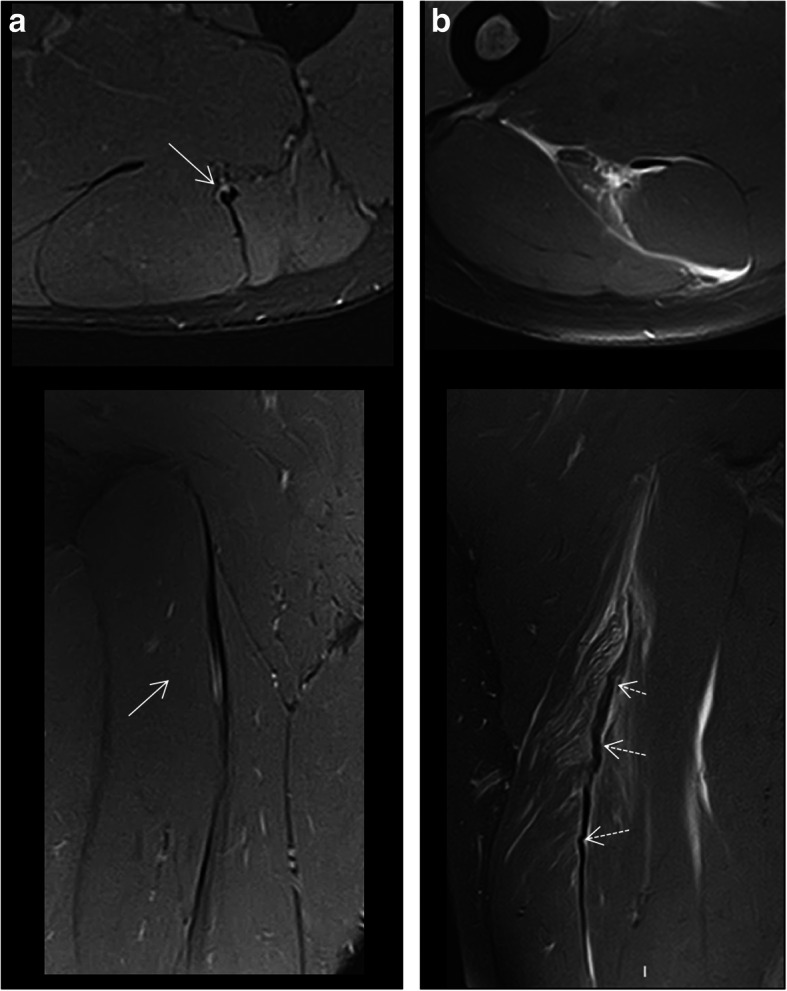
Coronal and axial MR images in fluid-sensitive sequences of the long head of the biceps femoris tendon. a Partial mixed disruption (arrows), predominantly longitudinal without loss of tendon tension. b Complete disruption with loss of tendon tension (wavy morphology, dashed short arrows), extensive interstitial edema, and intermuscular fluid
